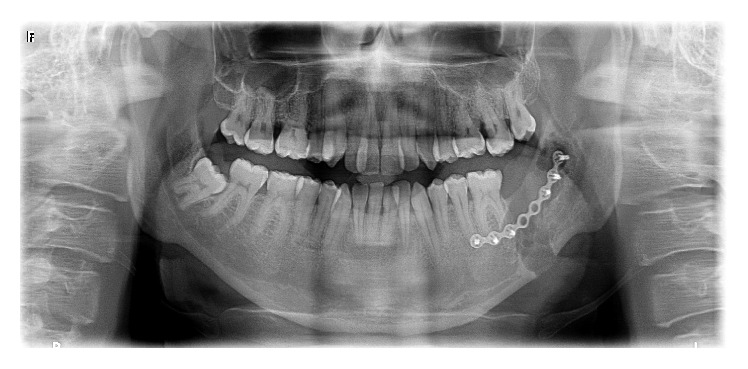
Postoperative panoramic radiography showing rigid fixation of the segments and complete removal of impacted teeth.
